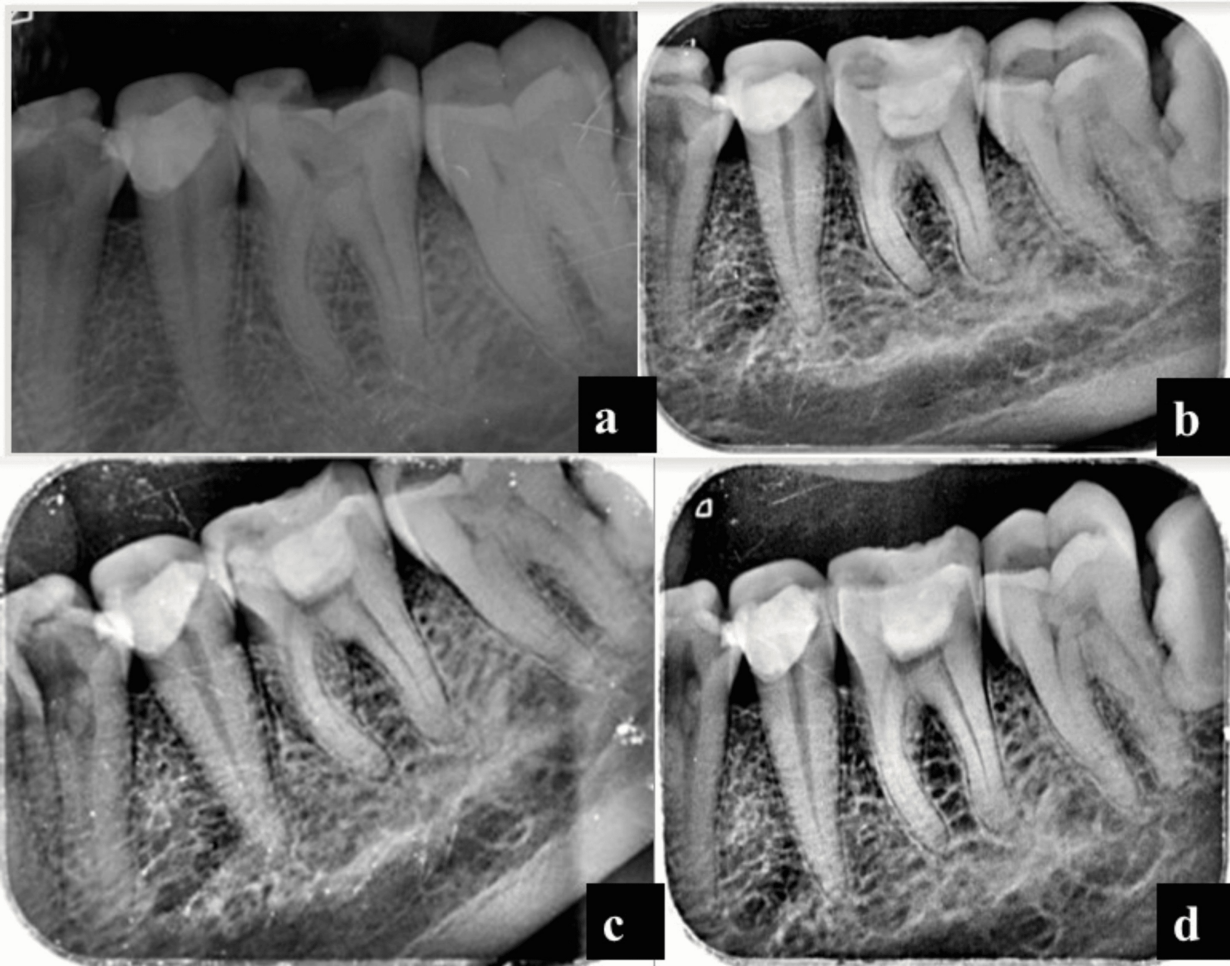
Preoperative radiograph (a); postoperative radiograph (b); 6-month follow-up radiograph (c); 12-month follow-up radiograph (d)
